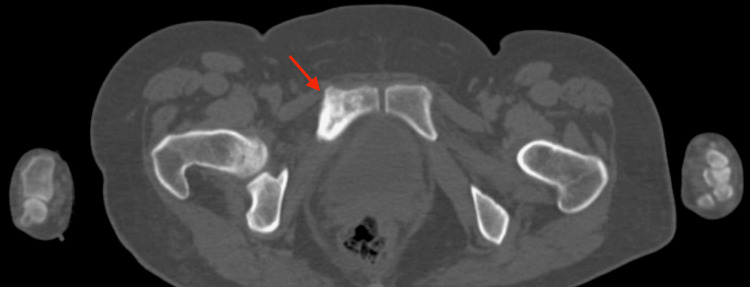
Abdominal computed tomography Blastic bone lesion in the right pubis
